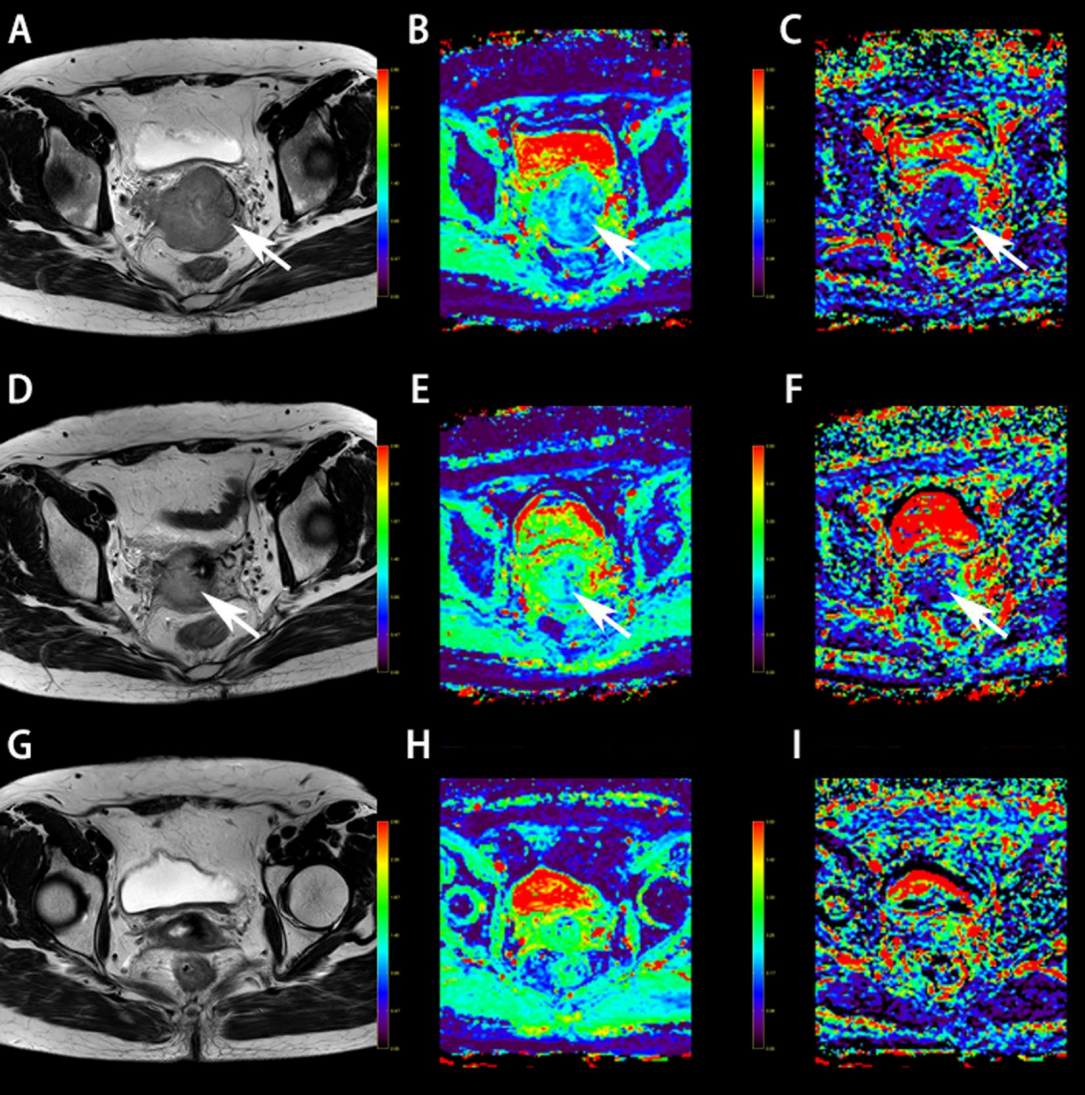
MR images of a 39-year-old female receiving CCRT for advanced cervical cancer (FIGO stage IIB). (A,D,G) Axial T2-weighted images at different time points in therapy (before CCRT, two weeks after initiation of CCRT and one month after initiation of CCRT), showing continuous decrease of the maximum diameter, at 5.07 cm, 3.37 cm and 1.15 cm, respectively; no residual lesion was observed one month after CCRT. (B,E,H) ADC_IVIM_ maps shows an increase in ADC_IVIM_ value during CCRT, as 1.07 × 10^−3^ mm^2^/s; 1.34 × 10^−3^ mm^2^/s and 1.50 × 10^−3^ mm^2^/s, respectively. (C,F,I) *f* maps shows an increasing value during CCRT, as 15.25%, 16.50%, and 27.70%, respectively. After a 28-month follow-up, this patient ultimately had a good prognosis.
